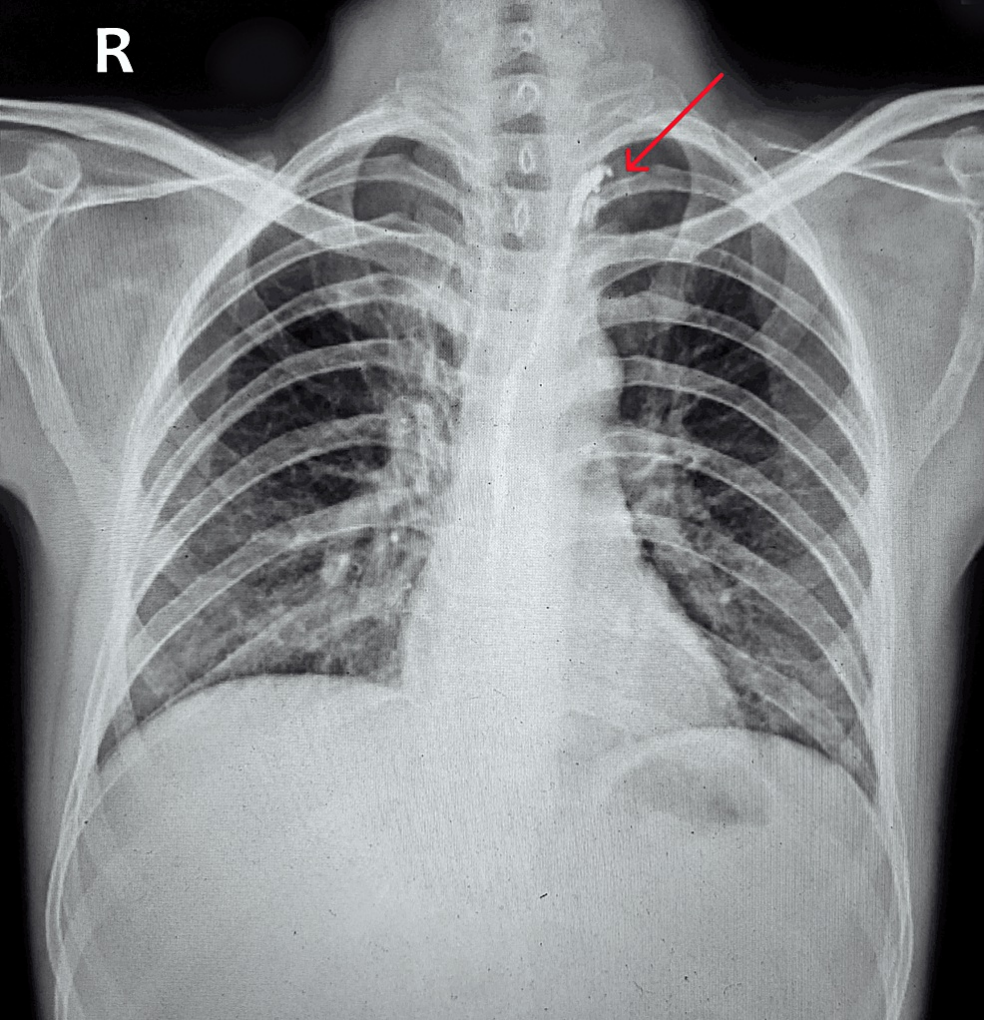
A chest x-ray anterior-posterior view post-discharge shows complete clearance of bilateral lung fields with bilaterally clear costophrenic angles indicating complete clearance of the chylothorax, with embolization of the thoracic duct (red arrow)
